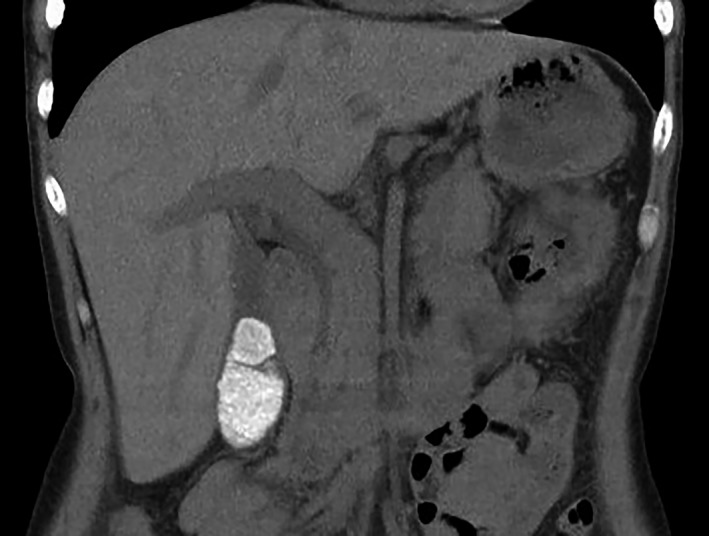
Abdominal contrast‐enhanced CT revealed a high‐density lesion in the gallbladder indicating ceftriaxone‐associated pseudolithiasis
